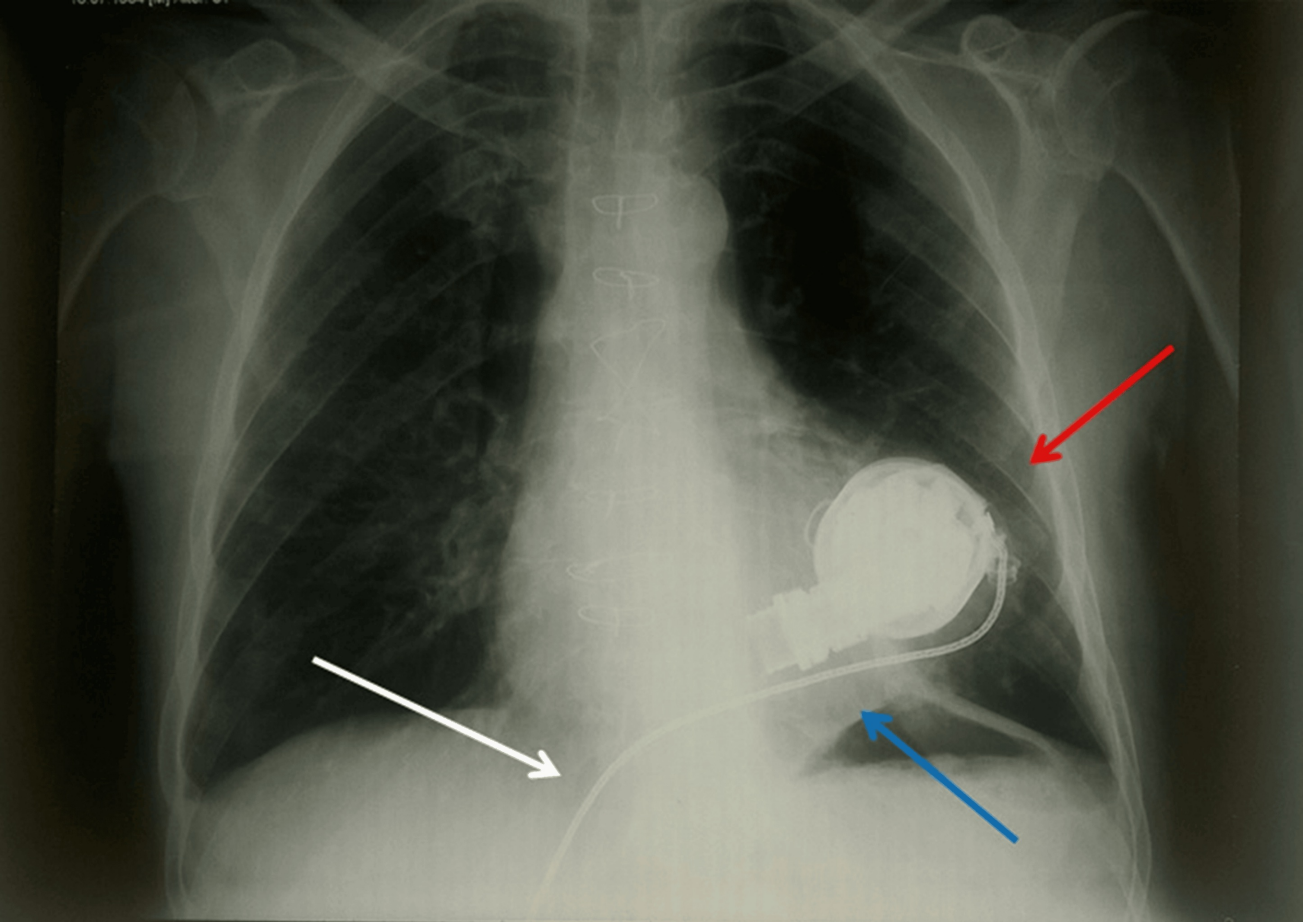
The X-ray shows the HM3 implantation at the apex of the left ventricle. The size is significantly smaller than previous generations, with less invasive implantation. Red arrow: magnetic levitation pump; blue arrow: left ventricle; and white arrow: drive line. This image was provided by the author (Bordoni Bruno) of this article. HeartMate 3 (HM3; Chicago, IL: Abbott)
